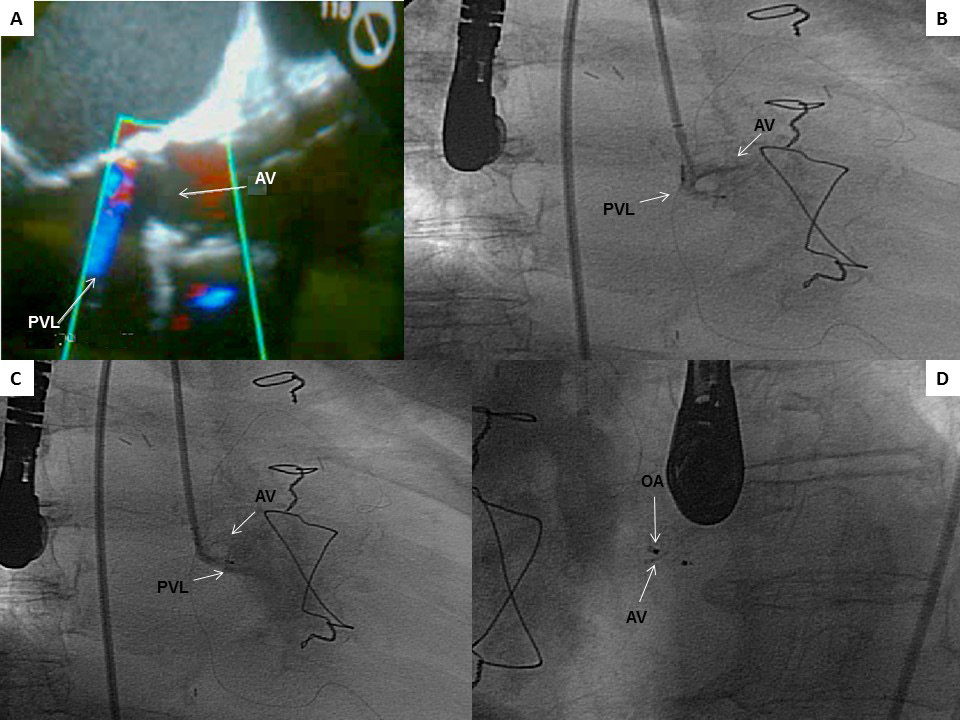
A) View of the aortic paravalvular regurgitant jet in a transesophageal
